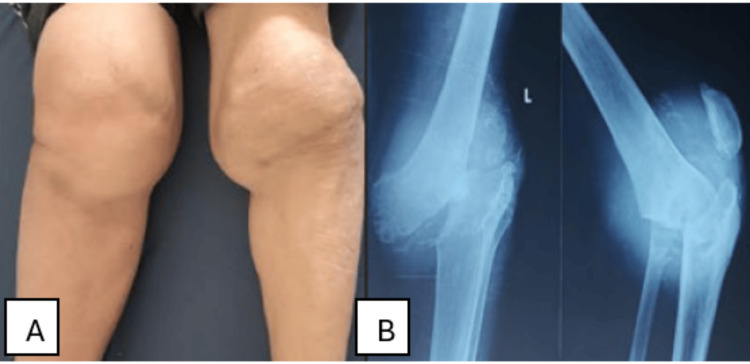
Imaging of Patient 1. (A) Clinical photograph showing the patient’s knees with structural deformation. (B) Lateral radiograph of the knees showing joint destruction and intra-articular fragments.
